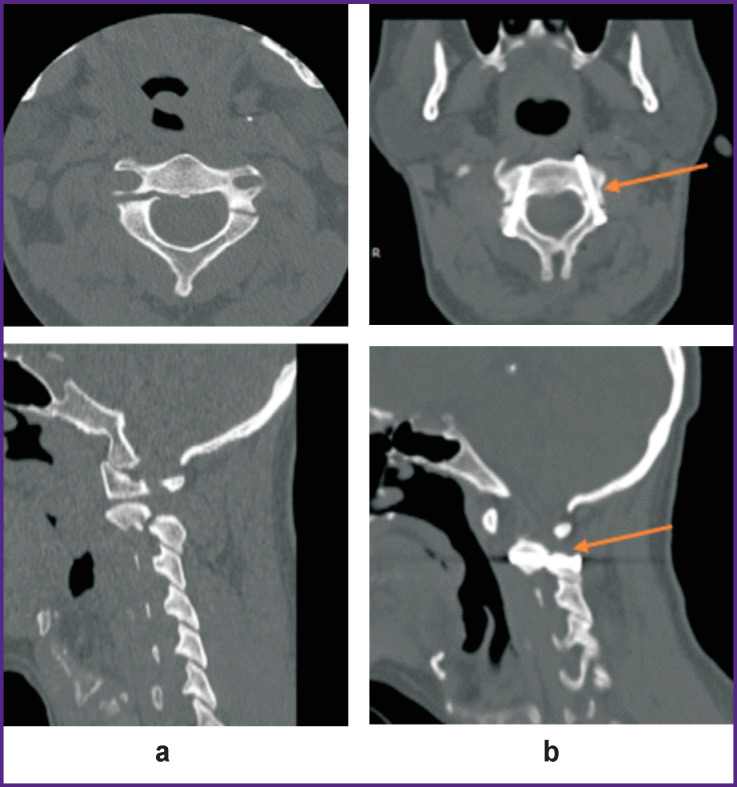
CT scans of the patient with traumatic spondylolisthesis of the C_2_ vertebra before (а) and after the operation (b) Arrows show compression screws
